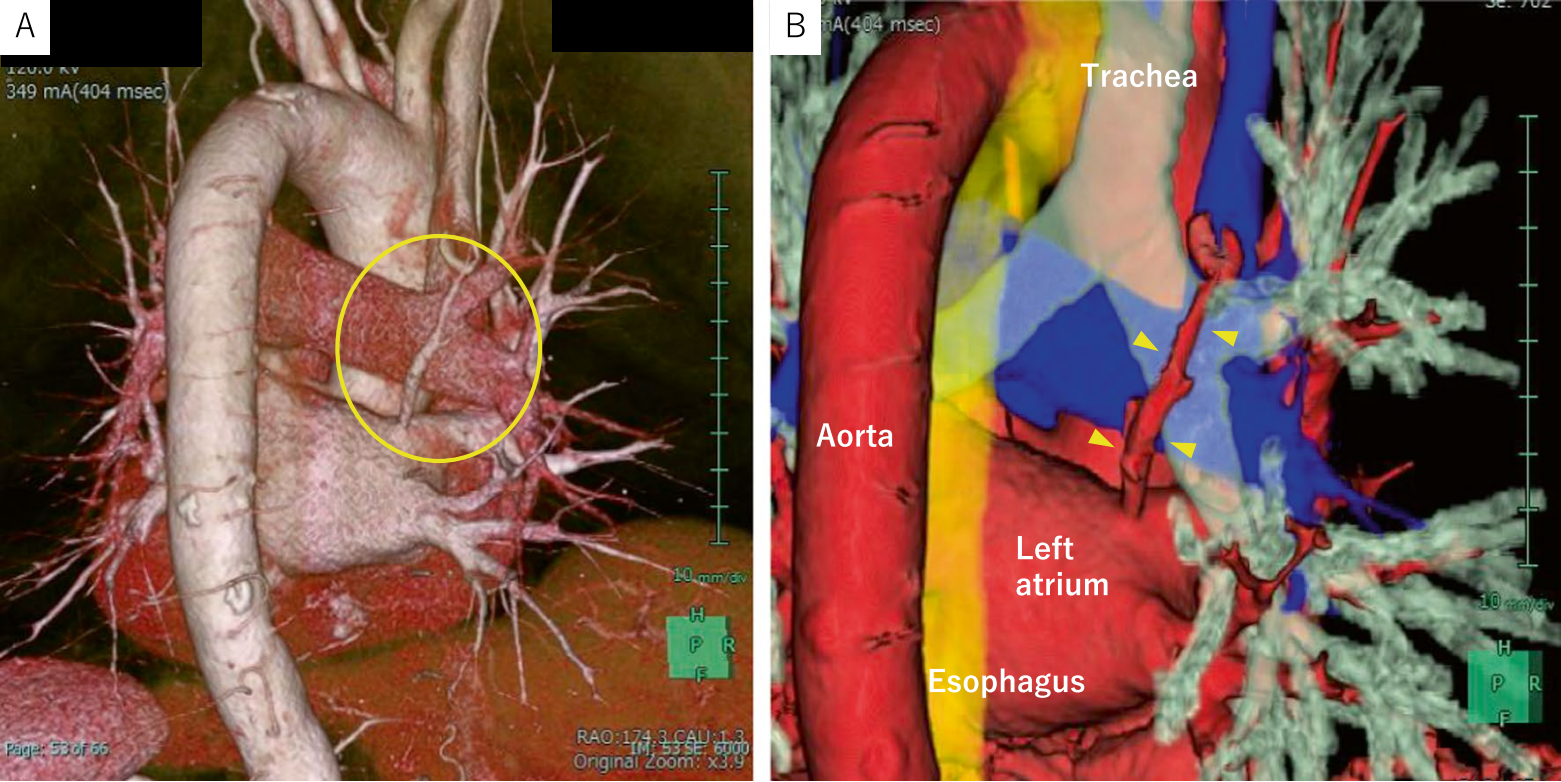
3D CT imaging. A, B 3D CT was performed preoperatively to confirm the 3D positioning. Arrowhead: The right pulmonary vein passing dorsal to the right main bronchus and flowing directly into the left atrium
